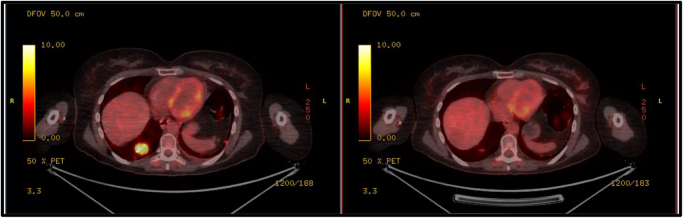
Baseline axial FDG-PET/CT image demonstrating intense hypermetabolic activity within mediastinal and hilar lymph nodes, consistent with metabolically active disease prior to initiation of trastuzumab deruxtecan (Enhertu) therapy.
